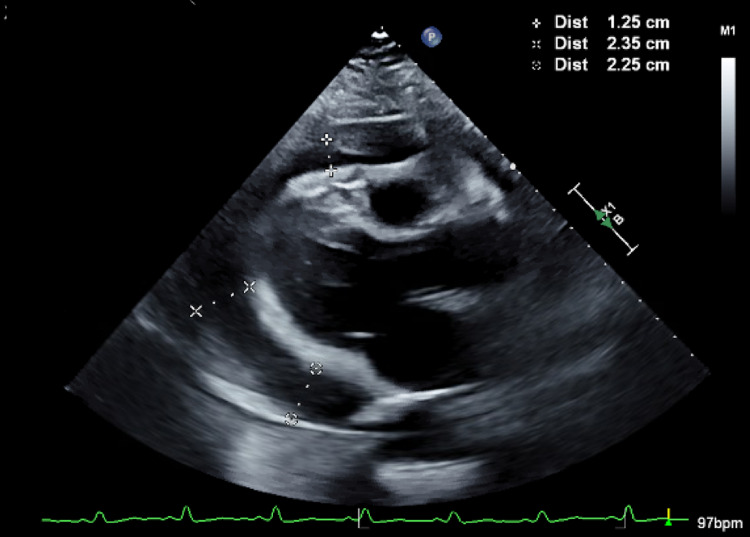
Transthoracic echocardiogram showing pericardial effusions with dimensions at the right upper corner
